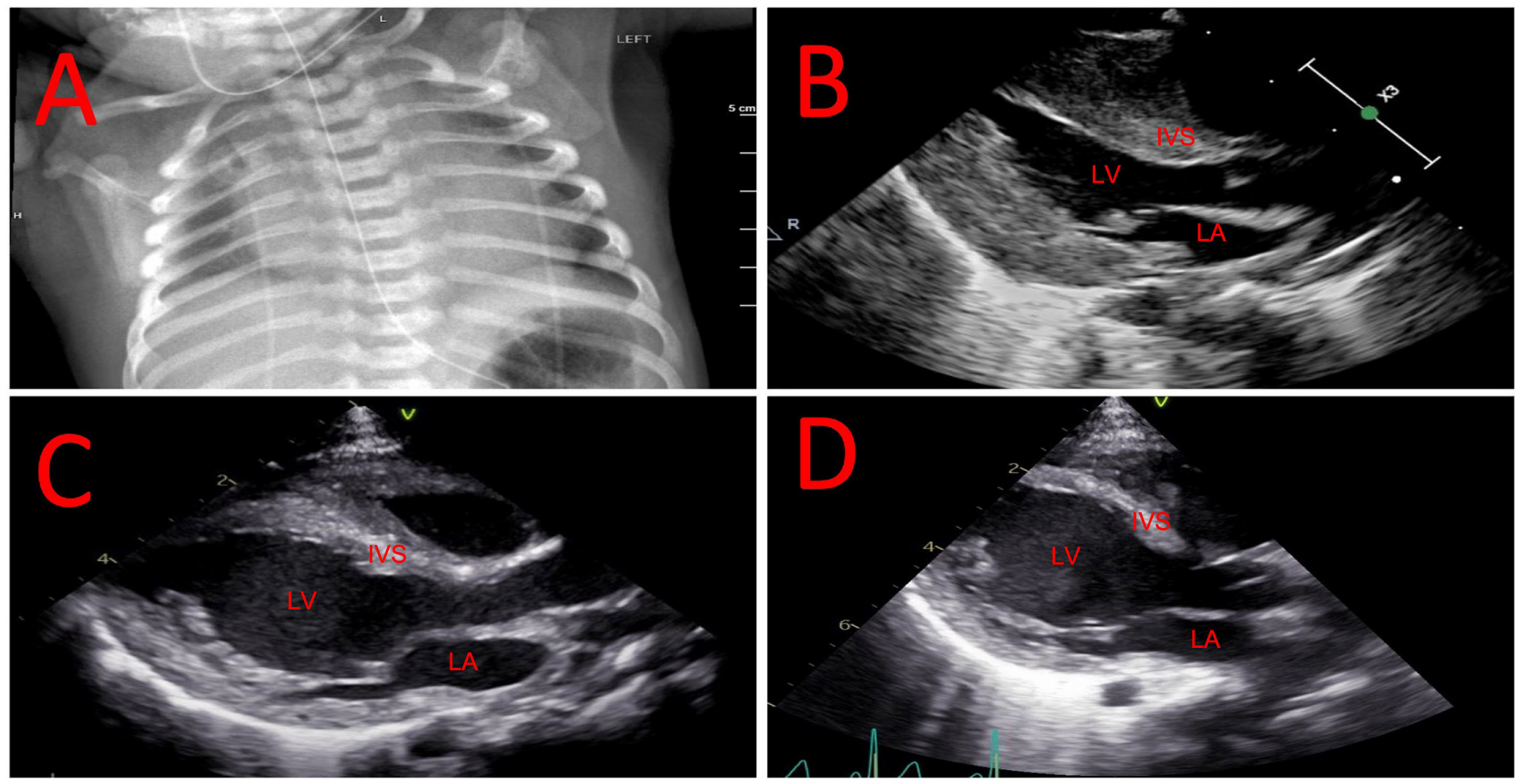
Evolution of cardiac hypertrophy and dilation. (A) Chest radiograph showing marked cardiomegaly in the neonatal period. (B) Echocardiogram showing hypertrophic left ventricle in the neonatal period. (C) Echocardiogram showing mixed left ventricular hypertrophy and dilation at 2 months of age. (D) Echocardiogram showing resolution of left ventricular hypertrophy with worsening of dilation at 7 months of age. IVS, interventricular septum; LA, left atrium; LV, left ventricle.
